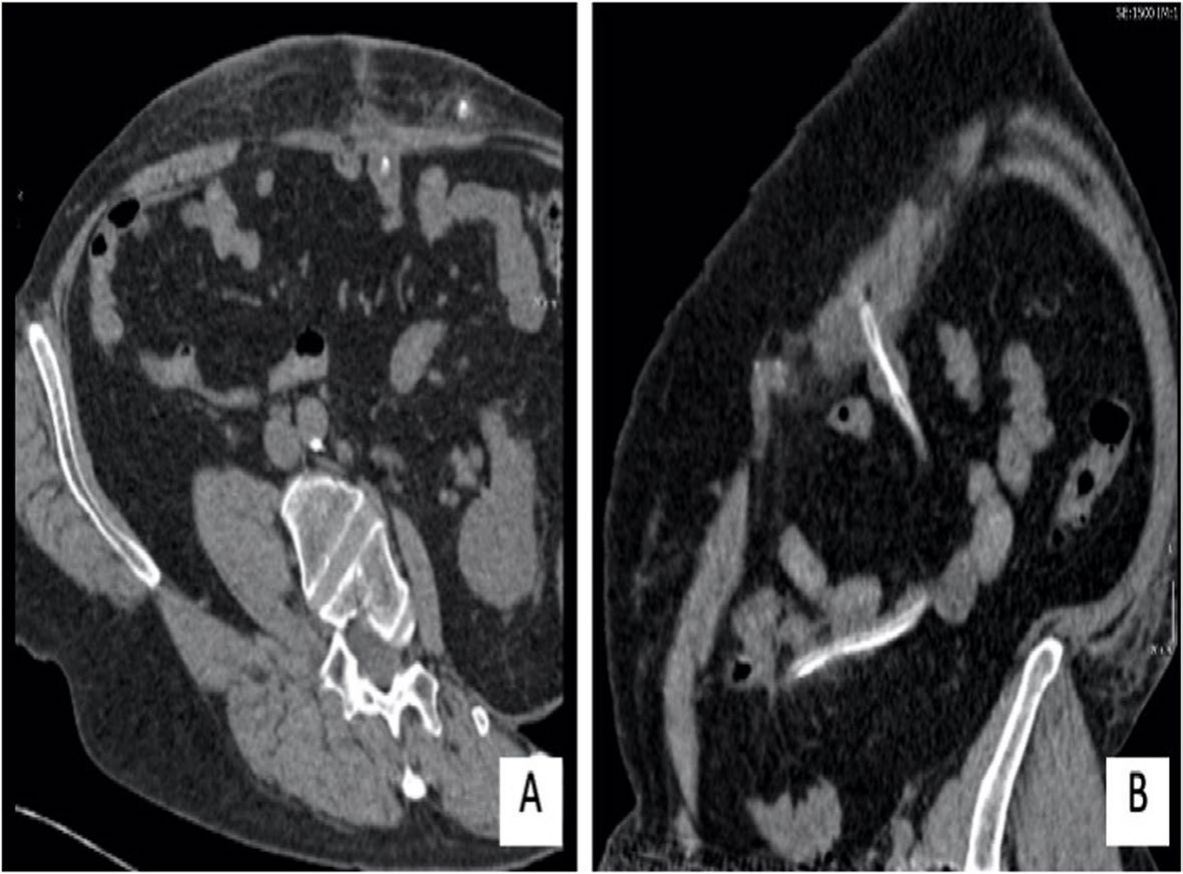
CT Scan of the abdomen: axial oblique (A) and coronal oblique (B) view showing the intraluminal trajectory of the dialysis catheter
